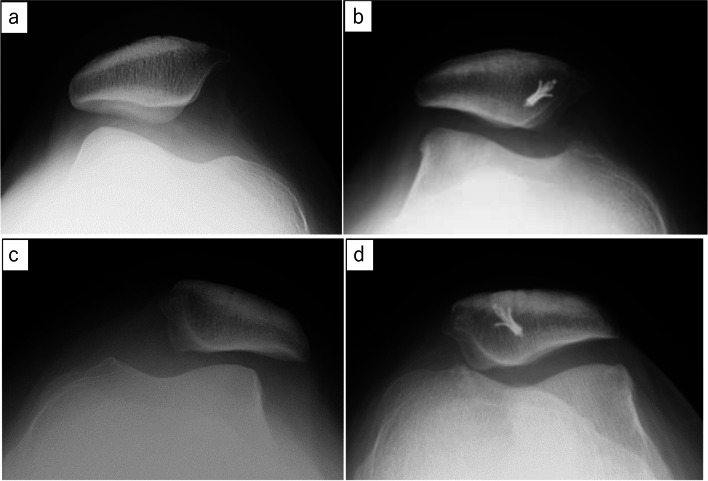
Representative pre and postoperative X-ray in the OA progression and non-progression case who underwent isolated medial patellofemoral ligament reconstruction (iMPFLR) and MPFLR with anteromedialization of the tibial tubercle osteotomy (MPFLR+AMZ). a Stage 0 of preoperative X-ray of a left knee at 16 years of age before isolated medial patellofemoral ligament reconstruction; b Stage 1 of postoperative X-ray 5 years following iMPFLR. c Stage 1 of preoperative X-ray of a right knee of a 35-year-old woman before MPFLR+AMZ. d Stage 1 of postoperative X-ray 7 years following MPFLR+AMZ
